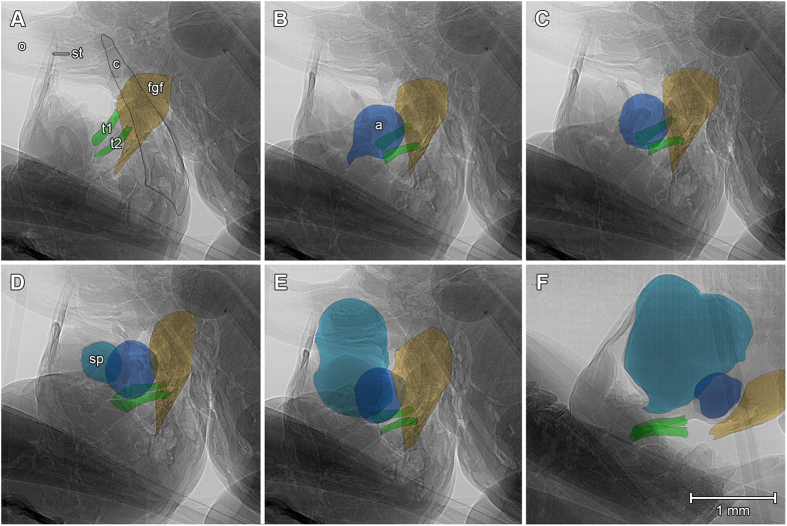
Radiography of male and female genital interaction during spermatophore transfer by a bushcricket mating couple. The images were extracted from the video S2, which was recorded at recorded at 25 fps, 3.6x magnifications, 3.06 μm pixel size. (A) During the spermatophore ejection the male’s cerci (c) help to keep the female’s and male’s genitalia in close contact. (B,C) The male’s titillators (t1 and t2, green) are pushed down on the female’s genital fold (fgf, yellow) during the ejection of the ampullae (a, dark blue). (D–F) While pumping out the spermatophylax (sp, light blue), the ampullae slide over the titillators and are attached on the female’s genital opening.
